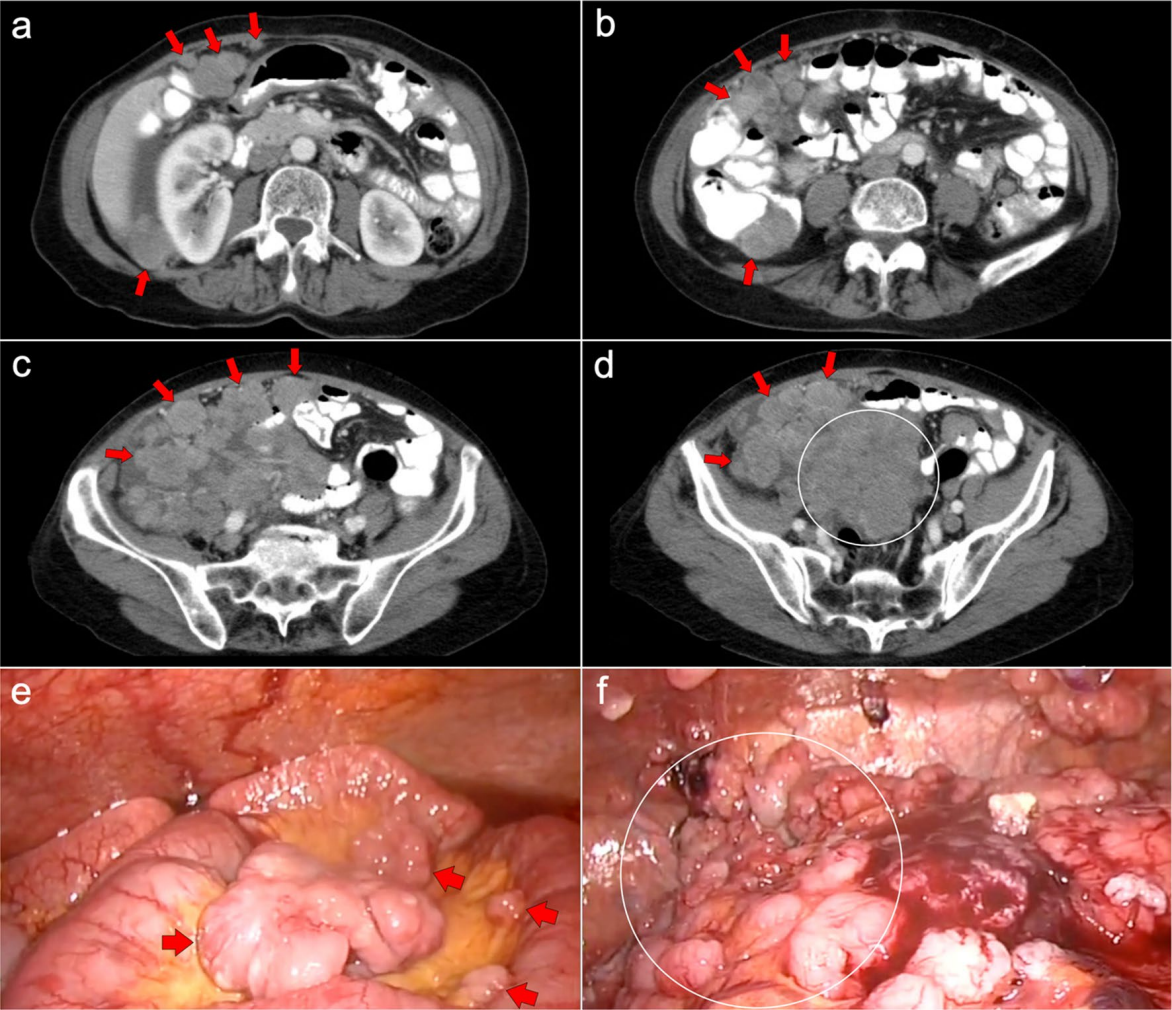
Malignant sarcomatoid peritoneal mesothelioma in a 69-year-old woman. a–d Axial CT images with positive oral contrast and intravenous contrast in the portal phase show a “dry type” malignant sarcomatoid mesothelioma, presenting with minimal ascites and multiple solid intraperitoneal lesions (red arrows) that converge into a bulky mass at the mesenteric root (white circle). e, f Laparoscopic images show several solid implants in the small bowel mesentery (e, red arrows) and multiple implants in the pelvis that converge in the inferior portion of the mesenteric root (f, white circle)
